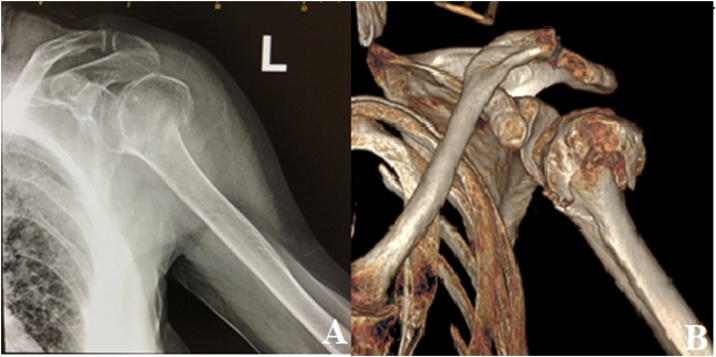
(A) X-Ray radiograph of the left shoulder showing a comminuted proximal humerus fracture. (B) CT 3D reconstruction of the left shoulder showing a Neer III displaced fracture of the humeral neck.
